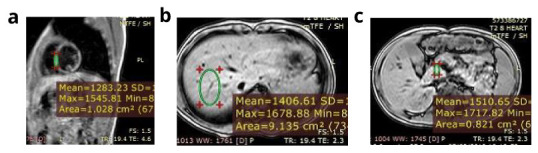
Magnetic resonance imaging of a patient with moderate cardiac iron overload, normal hepatic and pancreatic iron concentration. a) Calculated myocardial T2* equals 13.3 ms and myocardial iron concentration equals 1.91 mg/g (moderate cardiac iron overload).
